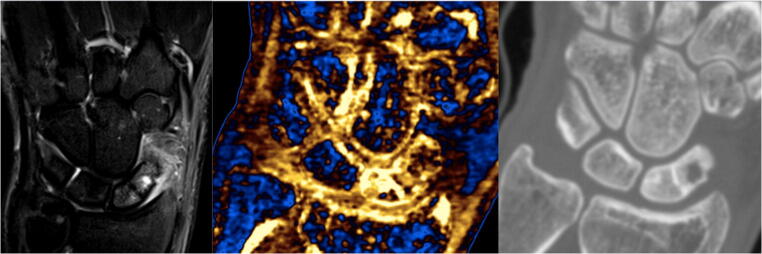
Diffuse acute scaphoid oedema. The STIR sequence of the MRI (left) and DECT with calcium/water filter (middle) showed diffuse scaphoid oedema. The oedema is highlighted in gold on the DECT with calcium/water filter image. The MRI also showed a distal pole fracture and a bone cyst in the proximal pole, which were also identifiable on the CT component (right)
